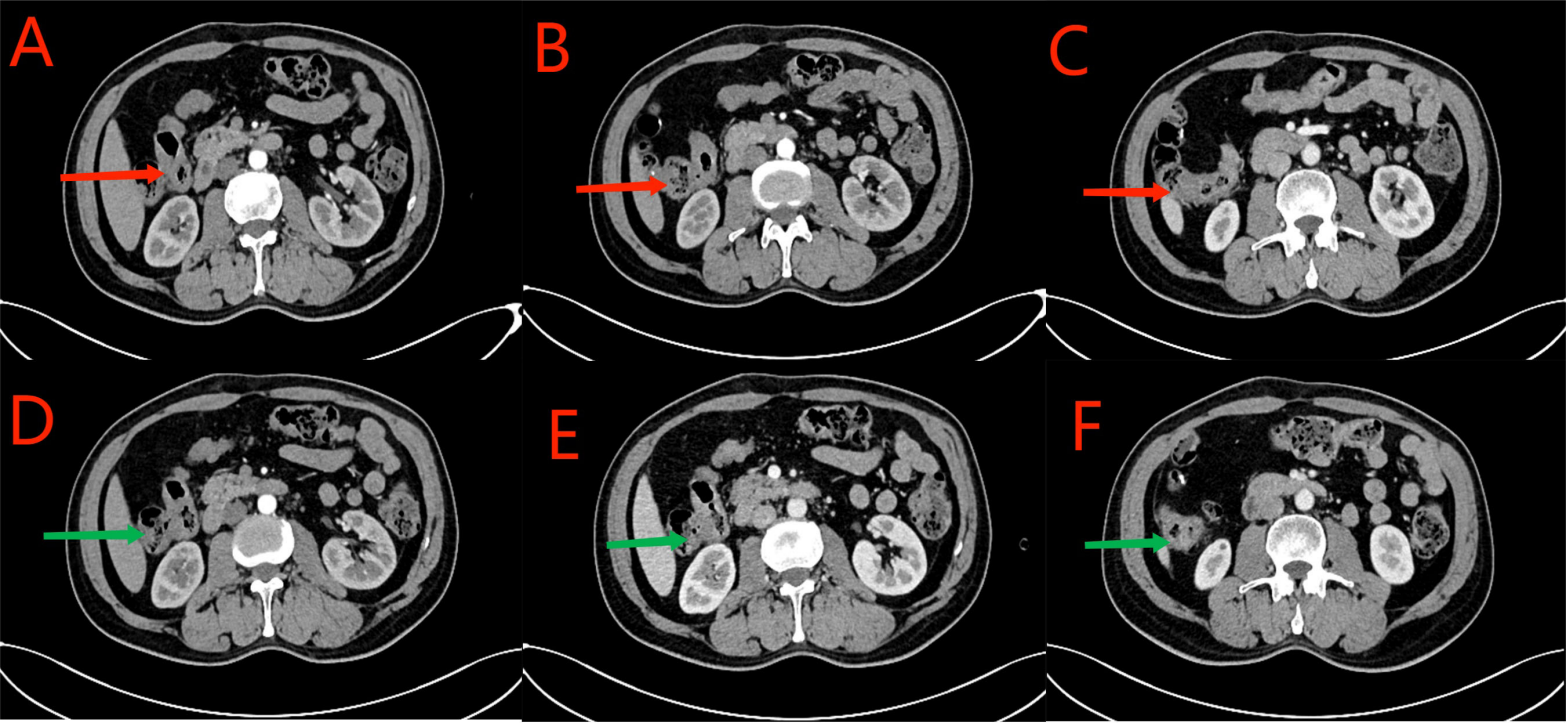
(A–C) CT scan conducted on September 27, 2023. Red arrows indicate local thickening of the anastomosis and distal intestinal wall; lymph nodes in the surrounding adipose space are shown. Based on the clinical data, no significant change was noted when compared with the CT scan findings of July 21, 2023. (D–F) CT scan conducted on December 26, 2023. Green arrows indicate local thickening of the anastomosis and distal intestinal wall; lymph nodes in the surrounding adipose space are shown. No significant change was noted when compared with the results of the CT scan conducted on September 27, 2023.
